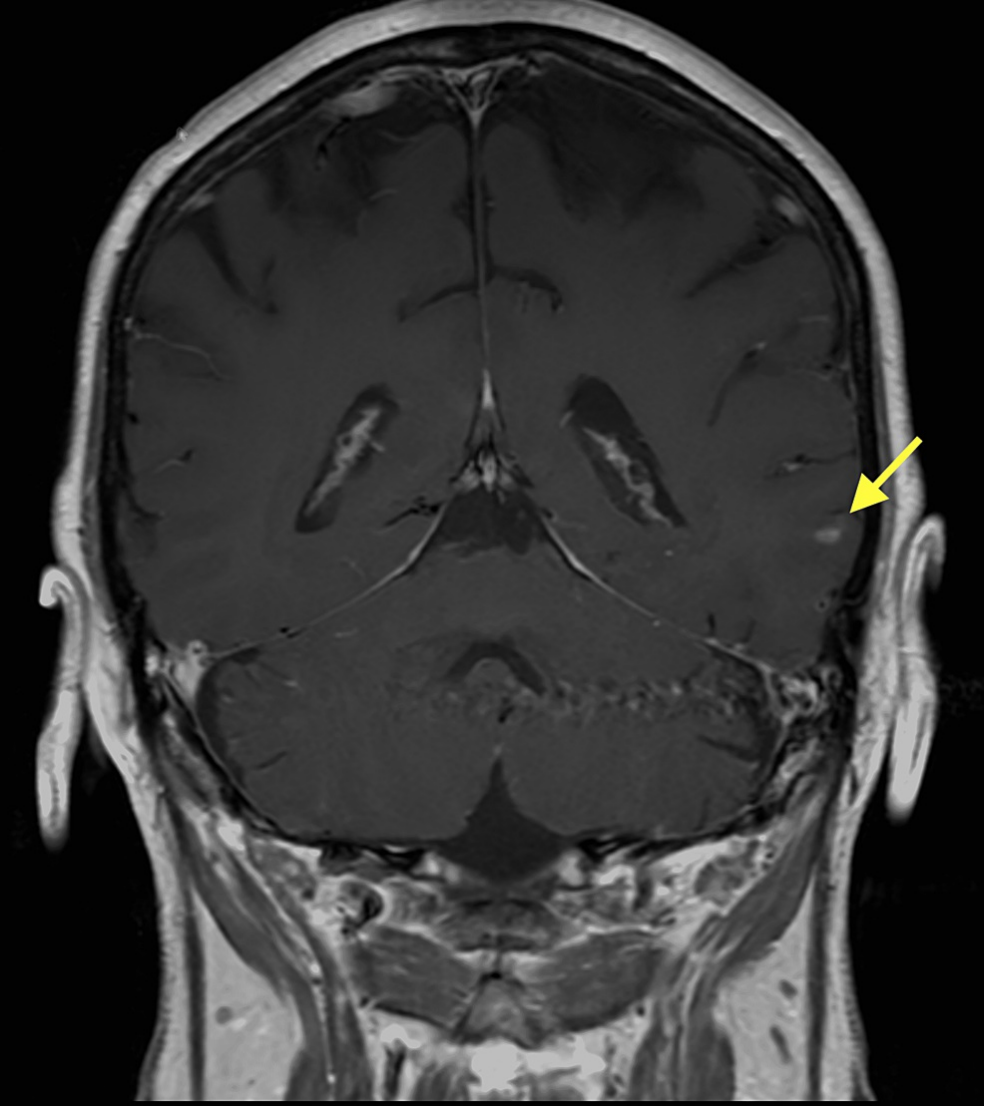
Brain MRI coronal T1 post-contrast image 12 days prior to admission. The yellow arrow shows stable enhancing focus lateral left temporal lobe from previously treated metastatic disease. MRI: magnetic resonance imaging
